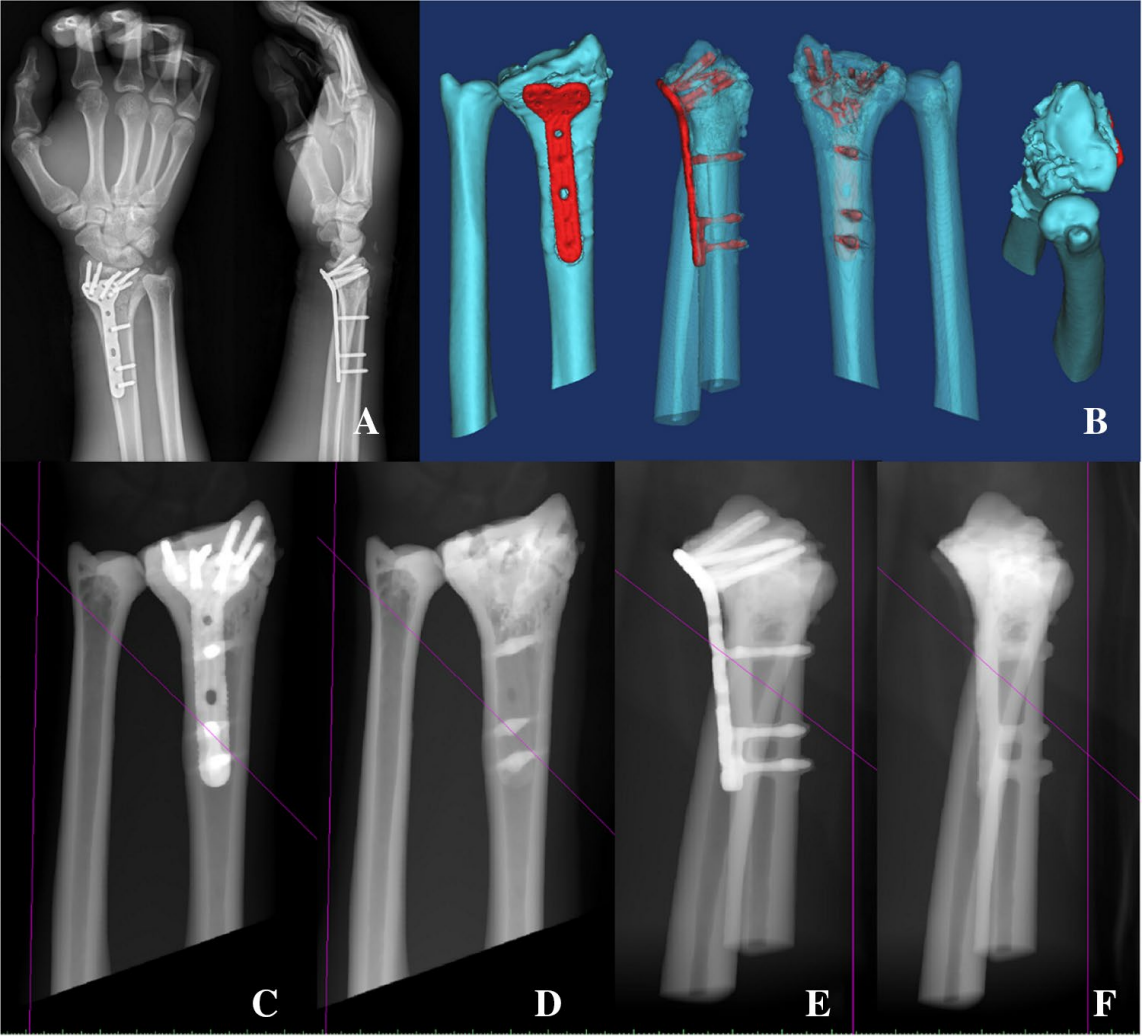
Typical real X-ray images (a, c–f) and virtual 3D reconstruction (b) demonstrated the final surgical effect (pink lines in c–f are system generated reference lines without any clinical implication)
